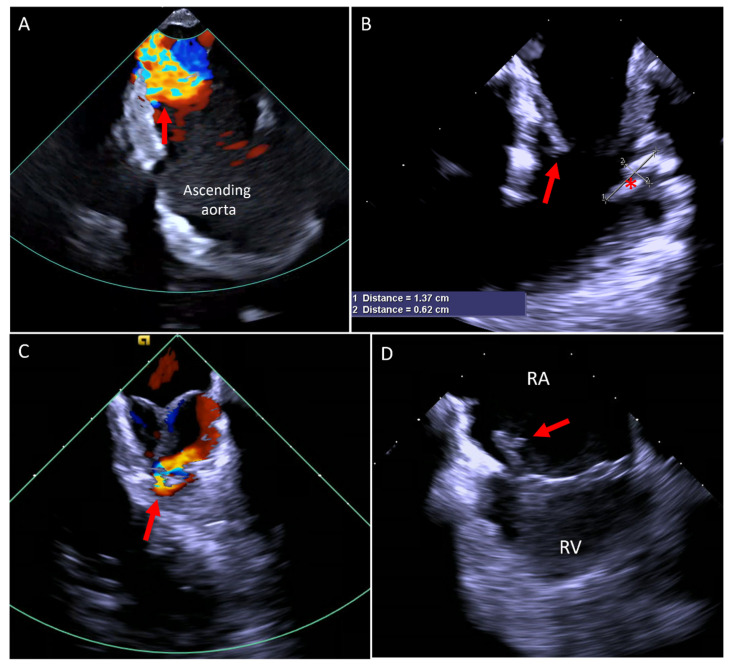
(A) Color Doppler image taken from the RVOT area, looking at the aortic valve showing severe aortic regurgitation (arrow). (B) RVOT imaging showing a thrombus (asterisk) distal to the pulmonary valve (arrow). (C) Color Doppler at the RVOT showing aortopulmonary fistula (arrow). (D) Two-dimensional image from the RA showing a thrombus on the pacemaker lead (arrow). RA, right atrium; RV, right ventricle; RVOT, right ventricular outflow tract.
